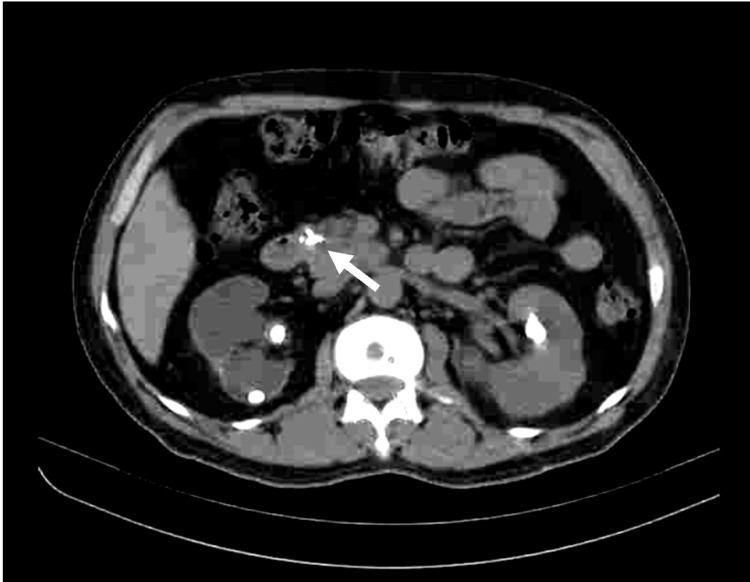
CT scan of the pancreas revealed chunky calcification (arrow) in the head of the pancreas. Calcification of the pancreas is an expected complication of long-standing hypercalcemia and may result in both exocrine and endocrine insufficiencies.
